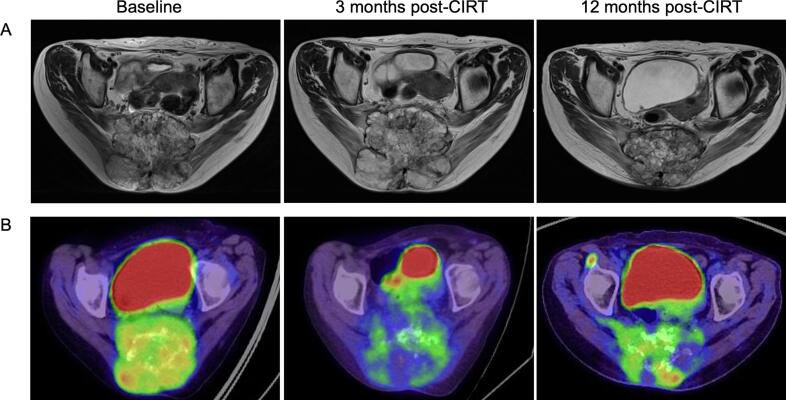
Representative case of tumor response in a patient with local failure. MRI and FDG-PET/CT images of a 72-year-old female with sacral chordoma treated with CIRT. A: MRI-T2WI, axial plane. The tumor’s LAD changed by +9% and −7% at 3 and 12 months post-CIRT, respectively. B: FDG-PET/CT-axial plane. The tumor’s SUV_max_ changed by −35% and +33% at 3 and 12 months post-CIRT, respectively.
